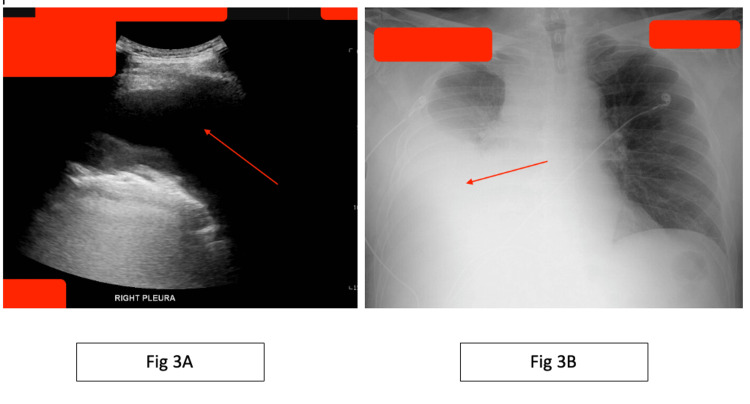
Chest ultrasound and X-ray of pleural effusion. Chest ultrasound on the right side showing pleural effusion (A). Chest X-ray showing right-sided pleural effusion (B).
